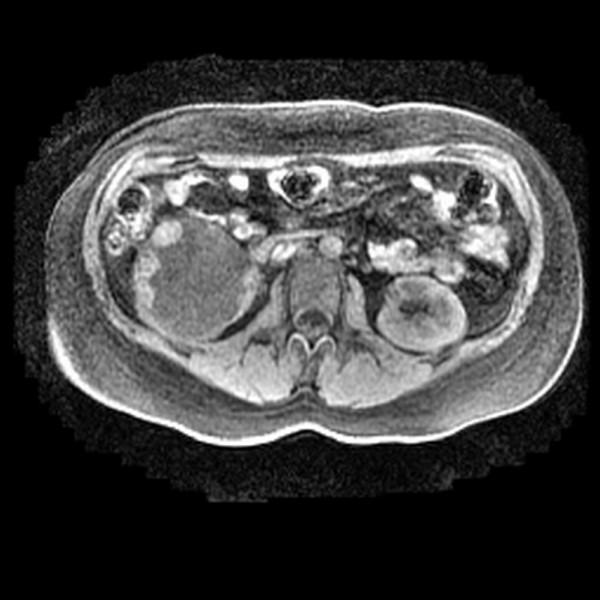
MRI scan showing unilocular cystic right renal mass with multiple mural nodule.
